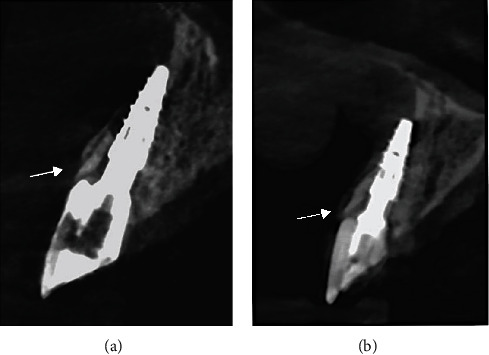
(a) At 6-month follow-up, CBCT images show the replanted root membrane in strict contact with the implant abutment and the bundle bone. The arrow indicates the portion of root not covered by bone. (b) At 48 months, CBCT images are compatible with the complete covering of the root membrane (see the arrow), as if there had been migration of the bone over all the root membrane surface.
